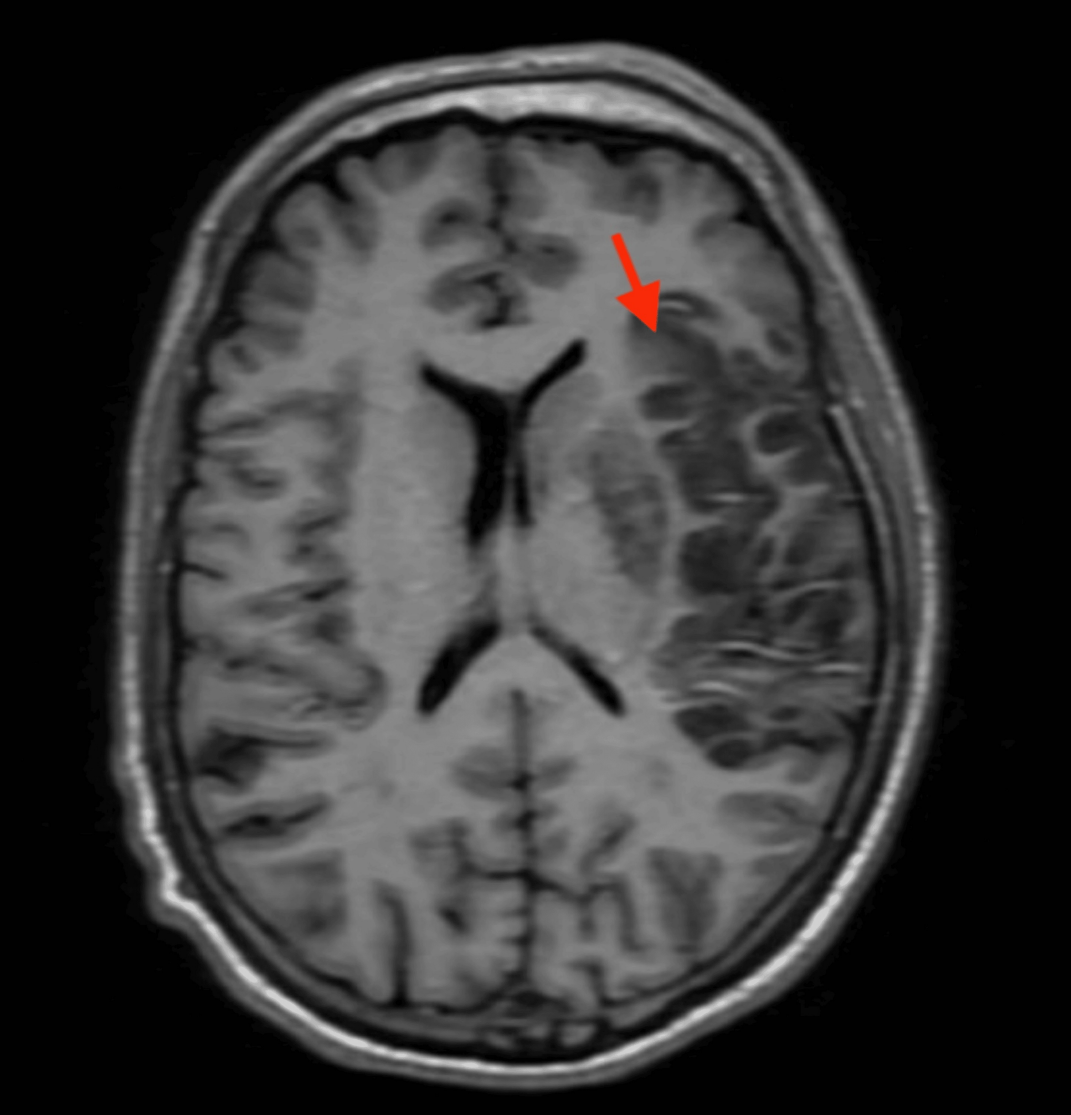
T1 FLAIR MRI of head shows area of hypo-intensity in the left middle cerebral artery distribution of the brain (red arrow) FLAIR - fluid attenuated inversion recovery
